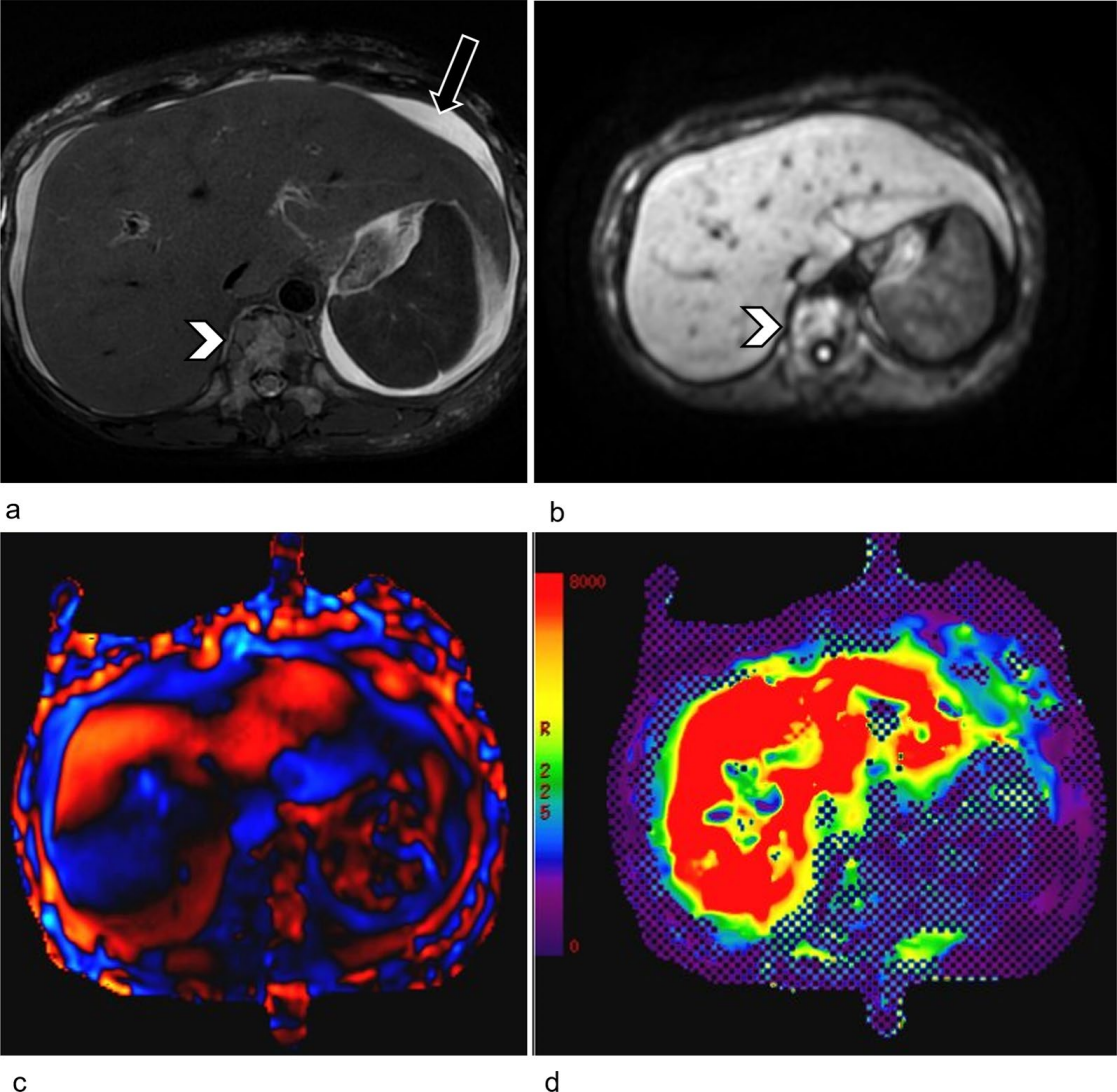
Metastatic ILC of the liver mimicking hepatic fibrosis in a 55-year-old female who presented with jaundice. She had a remote history of Stage 3C ILC more than 10 years ago. a Axial, fat-saturated, T2-weighted MR image and b diffusion-weighted image (b = 500 s/mm^2^) do not show any discrete hepatic lesion. The hepatic outline is smooth, with no morphological features of cirrhosis. Ascites is present (arrow). Bone metastases are also noted (arrowheads). c Wave images from the MR elastography acquisition at 60 Hz show shear waves with prolonged wavelength. d MR elastogram demonstrates severely increased mean shear stiffness in the liver, with corresponding quantitative color scale on the left of the image. Mean stiffness value is 15.6 kPa (normal < 2.93 kPa), in keeping with F4 fibrosis. The diagnosis of infiltrative metastatic ILC was confirmed on percutaneous liver biopsy
